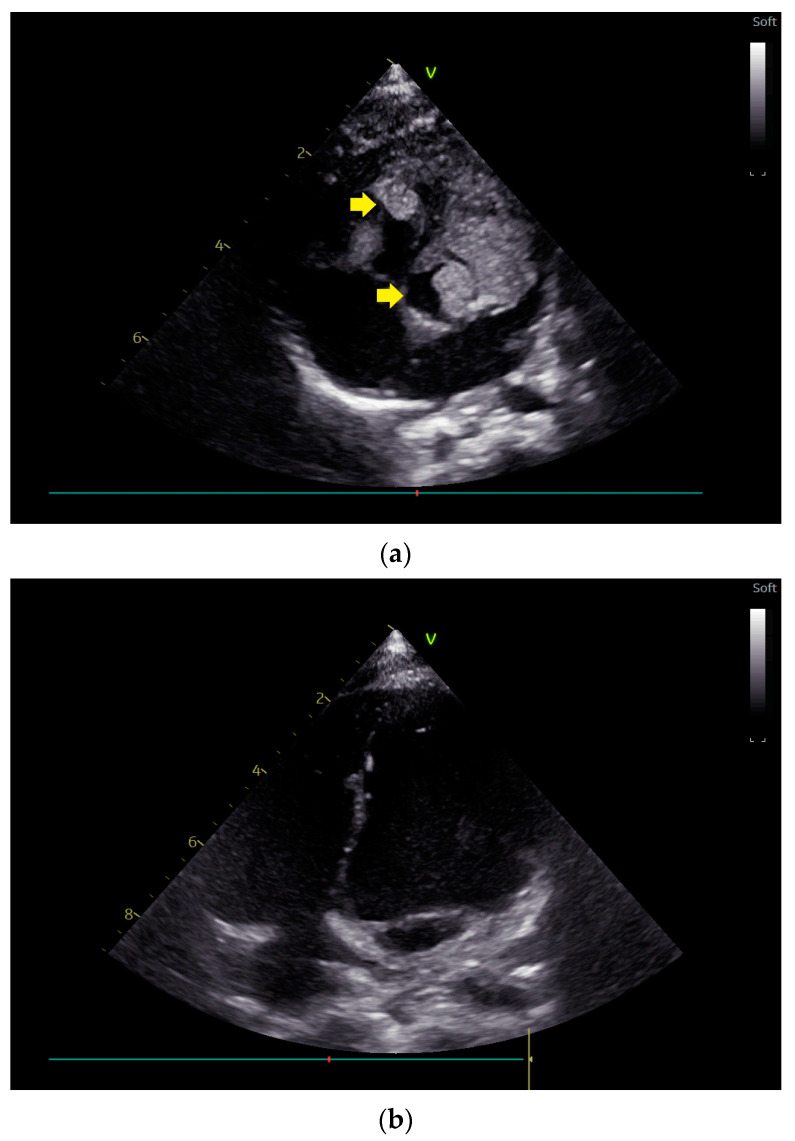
Transthoracic echocardiography showing multiple ventricular rhabdomyomas in the left and right ventricle (yellow arrows) in a neonate diagnosed with tuberous sclerosis (a) and their spontaneous partial regression at the age of 2 years (b).
